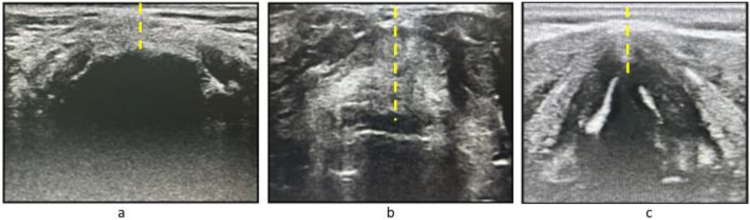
Ultrasound distances; (a) DSHB, minimum Distance from Skin to the Hyoid Bone, (b) DSE, Distance from Skin to the Epiglottis midpoint; (c) DSV, Distance from Skin to the Vocal cord anterior junction.
